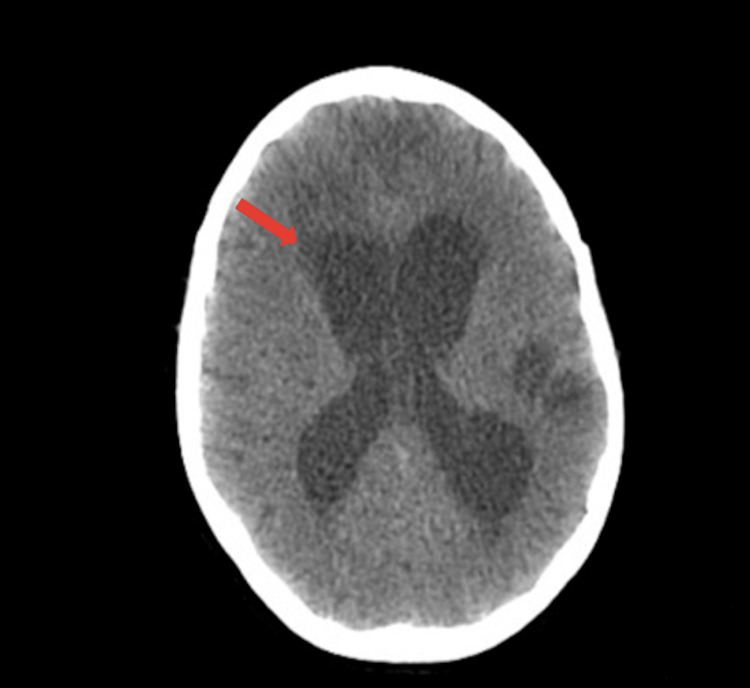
Cerebral CT scan showed persistent hydrocephalus.
